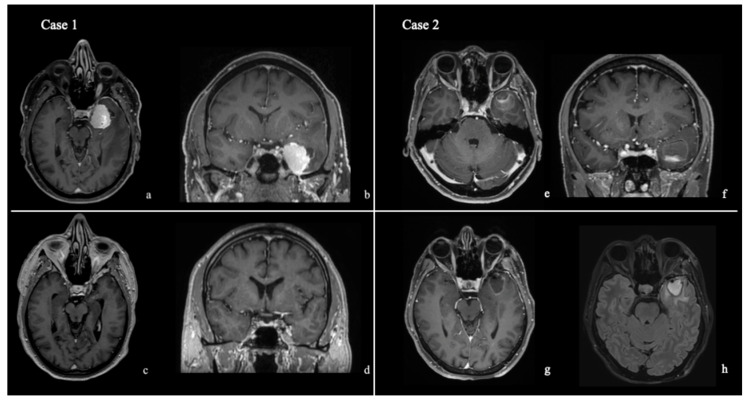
(Case 1) ref. [15]: A 64-year-old man presented with headache and anomia. (a,b) Preoperative contrast-enhanced T1-weighted MRI showing a left meningioma arising from the lateral wall of the cavernous sinus, associated with temporal lobe edema. Postoperative MRI (c,d) at 2-month follow-up demonstrating complete tumor removal (Simpson grade II). No postoperative visual, functional, or aesthetic deficits were observed. (Case 2): A 53-year-old woman presented with focal temporal seizures due to a temporal pole cavernoma. Surgical indication was established after documented rebleeding of the lesion. (e,f) Preoperative contrast-enhanced T1-weighted MRI in axial and coronal planes. (g,h) Postoperative MRI (contrast-enhanced T1-weighted and FLAIR sequences) showing complete lesion removal, with no evidence of orbital displacement or postoperative orbital edema following the TELKA. No postoperative visual or functional deficits were observed.
